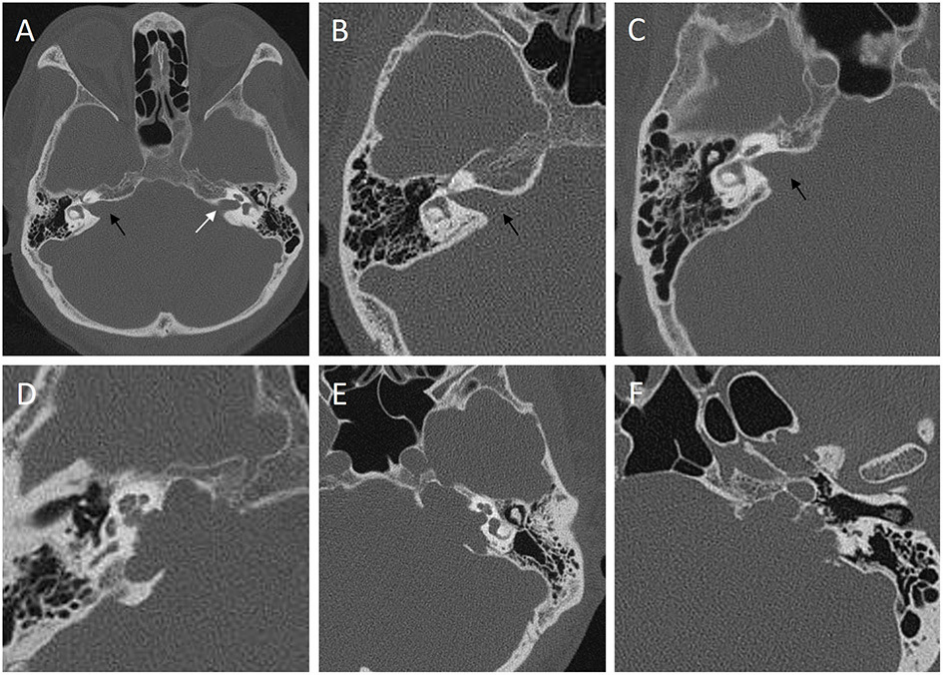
Classification of internal auditory canal (IAC) into regular type (A–C) and irregular type (D–F) according to the preoperative thin-slice CT bone window image of the temporal bone of the lesion side. (A) the right IAC is horn-shaped damaged type (black arrow) caused by a VS compared with the normal side (white arrow). (B) no expansion of the IAC (black arrow). (C) horn-shaped damaged type (black arrow). Curved damaged groove (D,E) or angled damaged groove (F) of the IAC wall could be observed in irregular IAC type.
